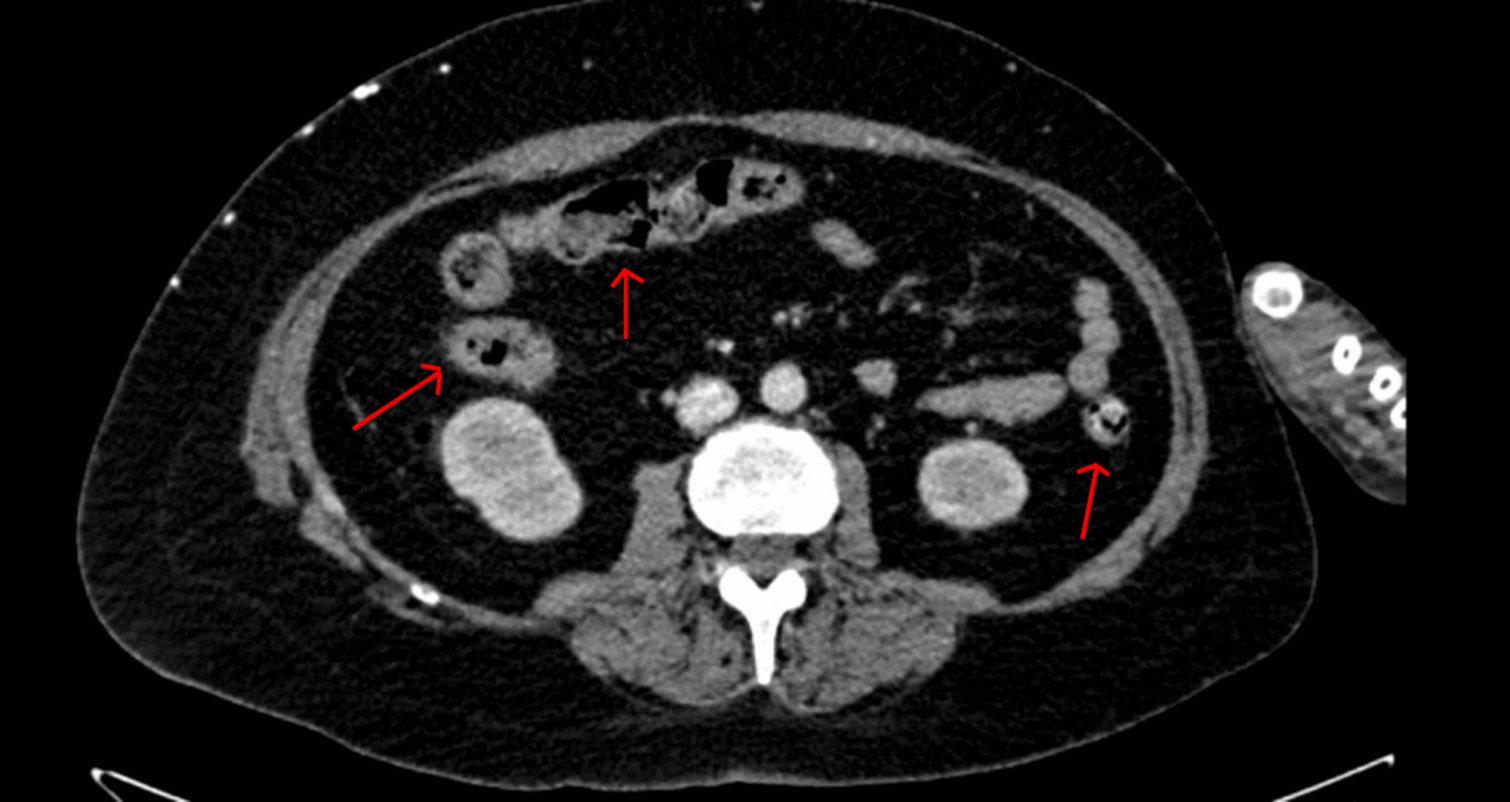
Repeat CT scan abdomen and pelvis Resolution of colitis
